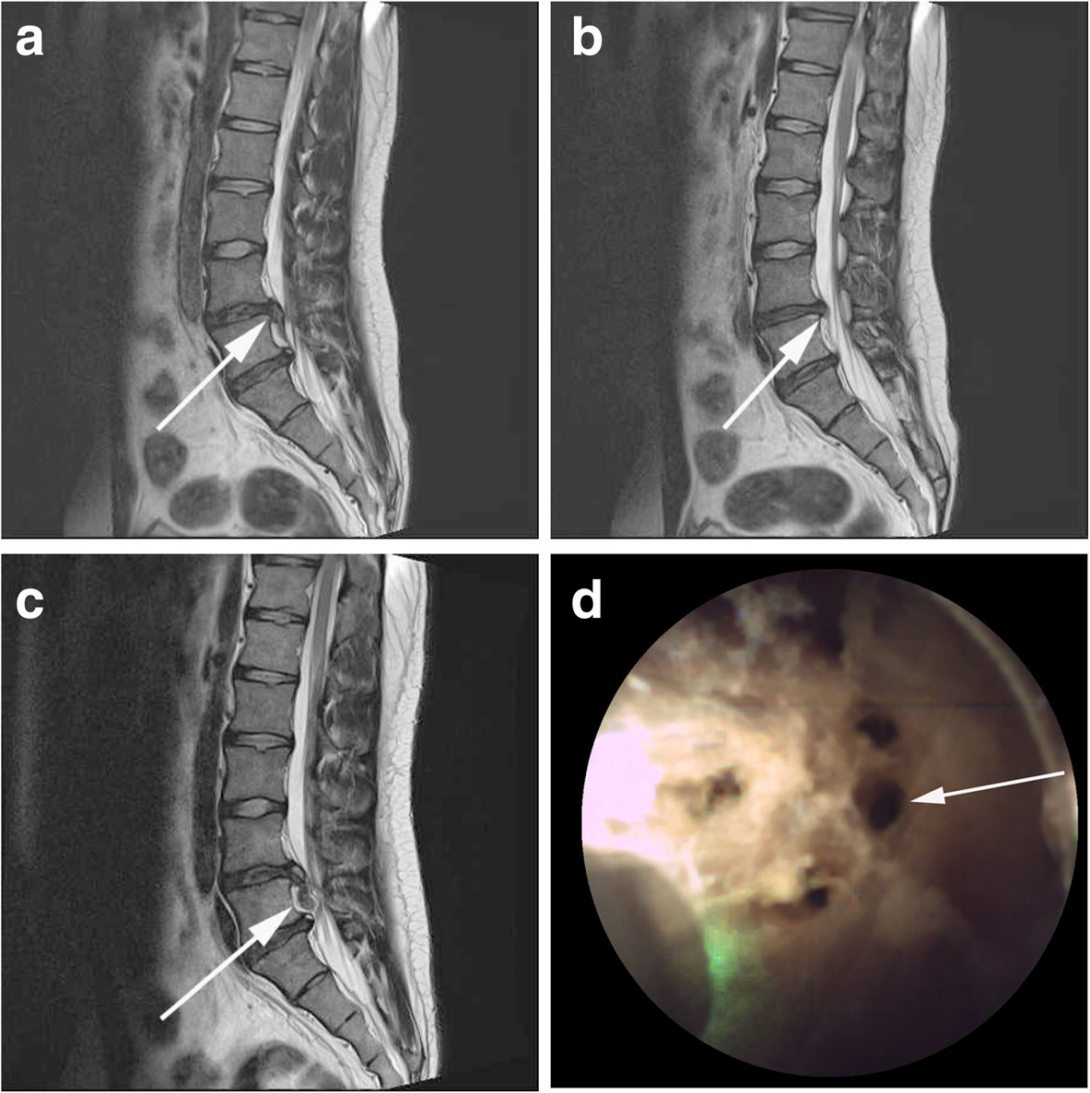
a Preoperative sagittal MR image showing a ruptured L4-5 disc with downward migration. b Sagittal MR image obtained 2 weeks postoperatively, showing decompression of the disc. c Recurrence of the disc rupture. d Epiduroscopic image showing multiple disc ablation holes. These multiple holes were likely to be the origin of the recurrence of the disc herniation
